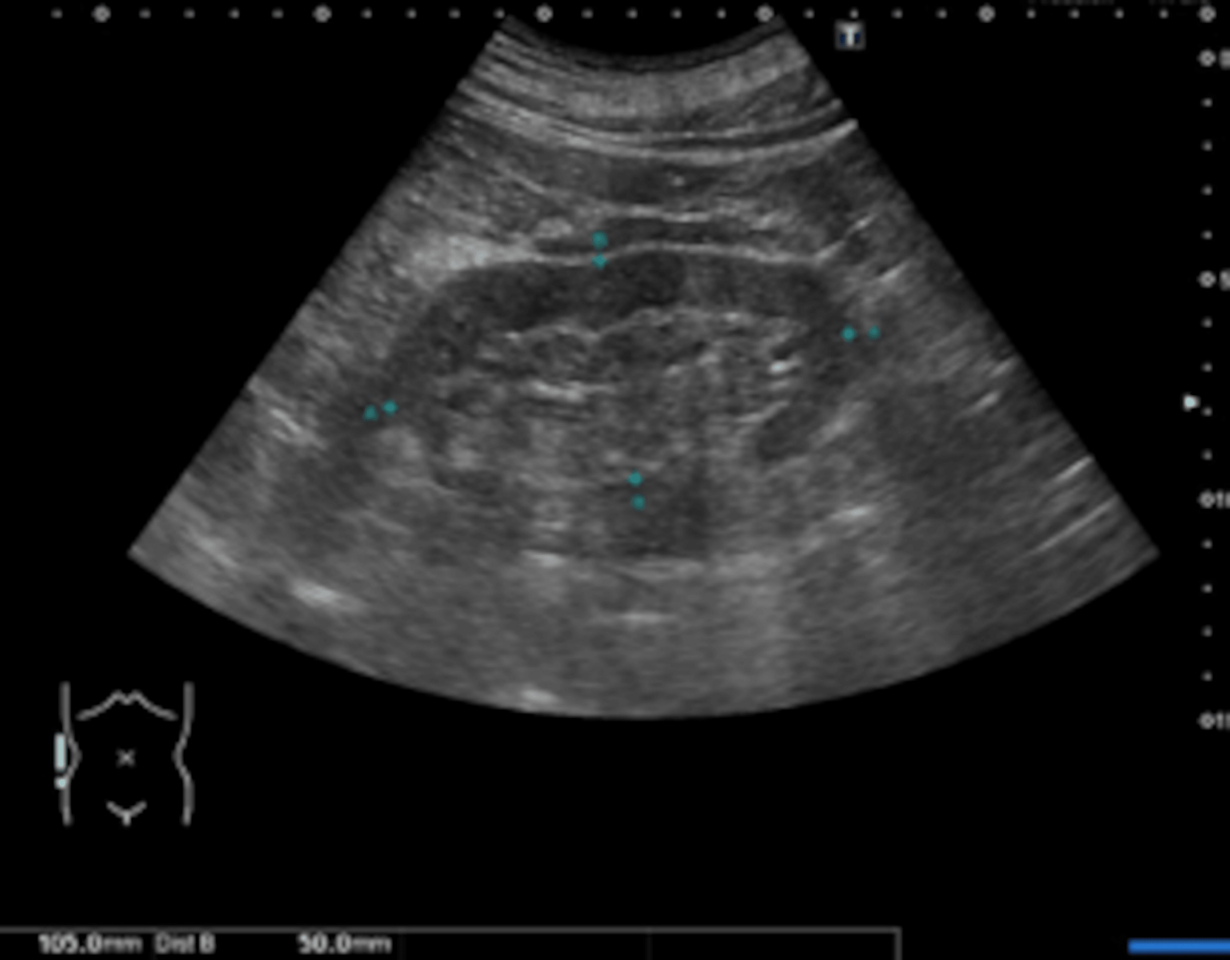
Right renal ultrasound findings. Renal ultrasound demonstrates irregular morphology with mild atrophy of the right kidney, which measured 105 mm in length and 50 mm in width, with no evidence of hydronephrosis.
